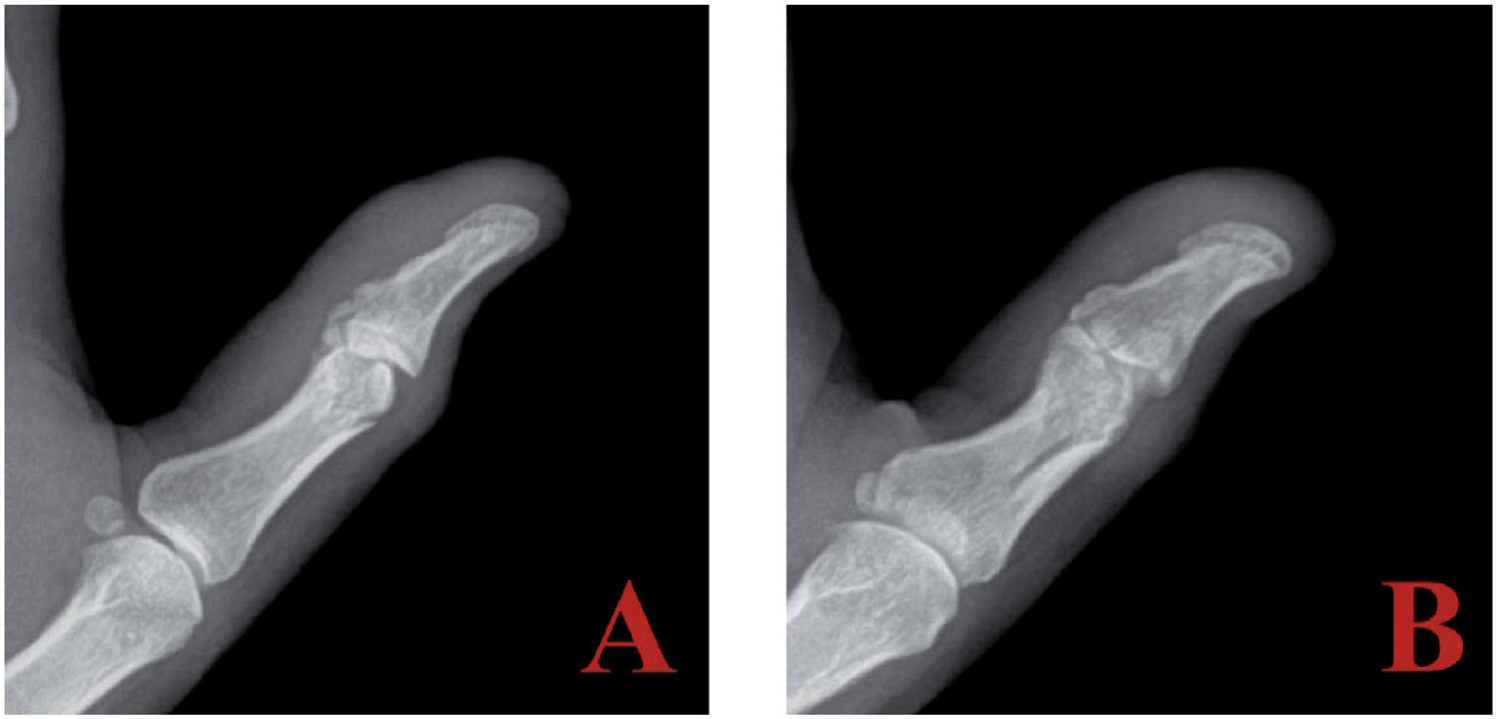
Plain radiograph lateral and oblique (A, B) of thumb two months post injury indicate early HO developing at the IP joint.
